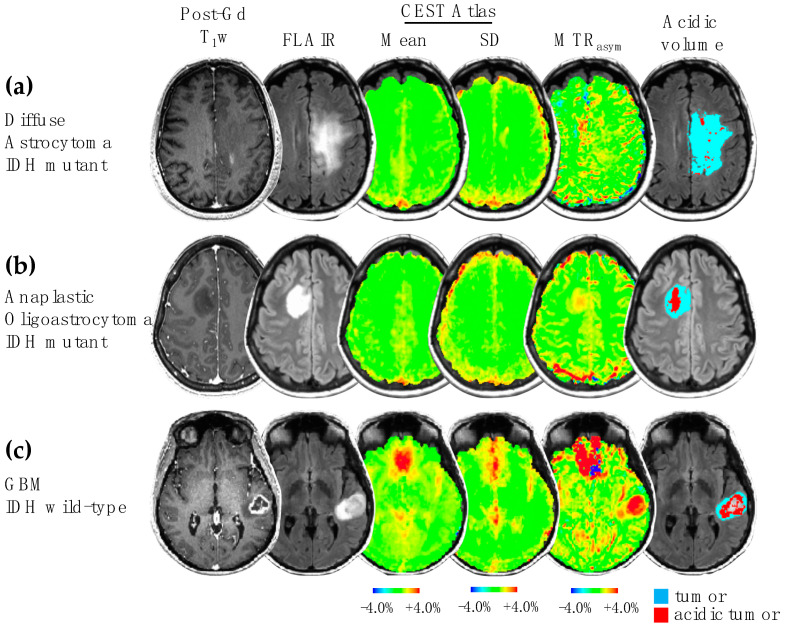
Examples of acidic volume determination with CEST atlas. Three patient examples with glioma grade II (a), grade III (b), and grade IV (c) are demonstrated, showing post-contrast T_1_-weighted image, FLAIR image, CEST atlas, MTR_asym_ at 3.0 ppm, and the acidic volume within the tumor. The high MTR_asym_ in the frontal brain region in patient (c) was due to an artifact caused by large B_0_ inhomogeneity in this region. SD: standard deviation.
